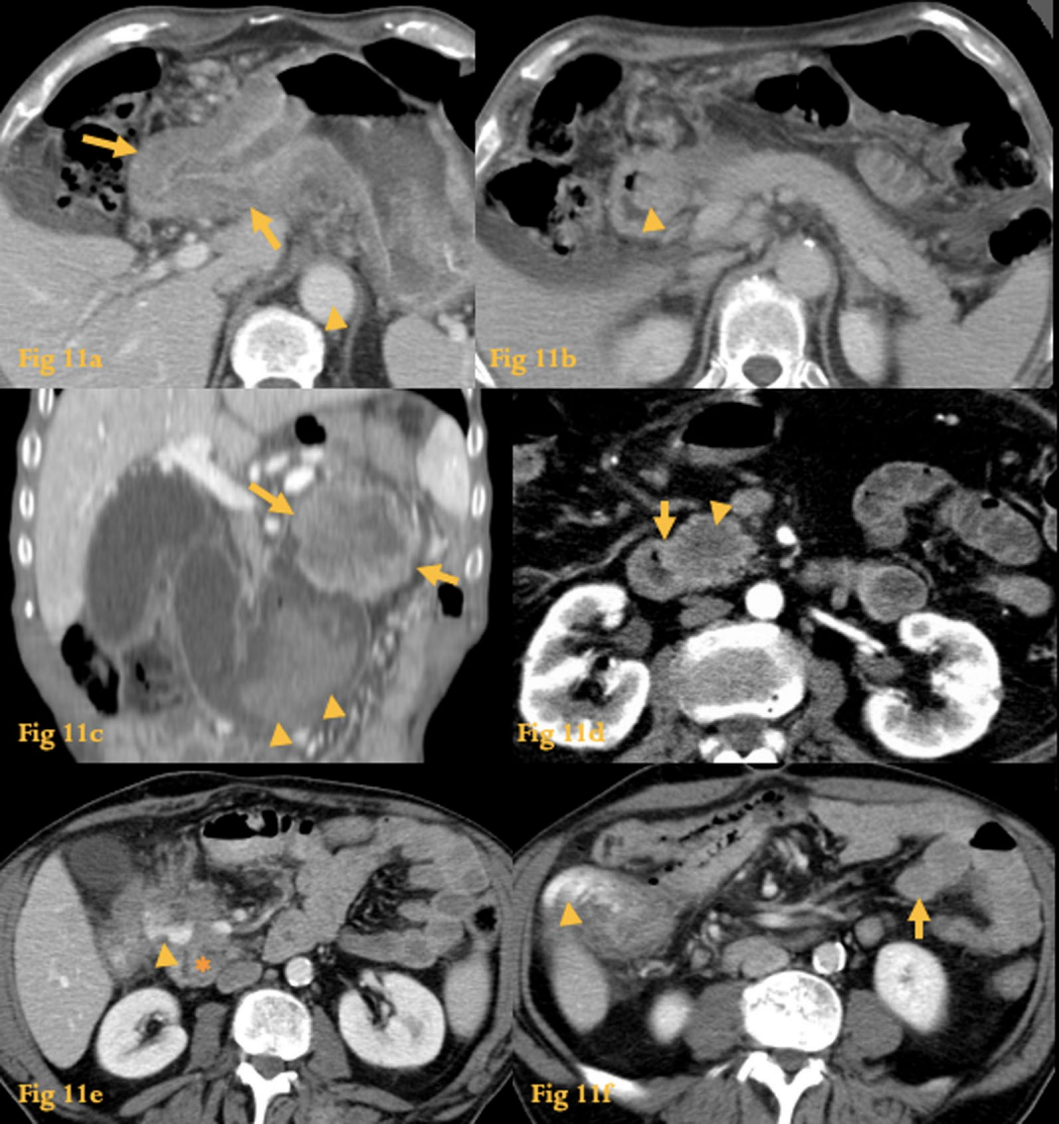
Extension from adjacent tumor. Abdominal CT (a, b) shows circumferential wall thickening of the gastric antrum (arrows) extending through the pylorus into D1 and D2, where a polypoid-like lesion (arrowhead) narrows the lumen. CT of another patient (c) shows evidence of gastric adenocarcinoma (arrows) with duodenal invasion and hemorrhage (arrowheads) inside the duodenal lumen. Abdominal CT of other patient (d) shows a hypodense soft-tissue density mass (arrowhead) which was a pancreatic adenocarcinoma with invasion of the medial wall of D2 (arrow). Abdominal CT of a patient with a neoplasm of the hepatic flexure of the colon (e, f). An undefined mass apparently involves the duodenum (asterisk) and the colon. A small amount of positive oral contrast agent (arrowhead) is present within the mass. An image obtained caudally (f) shows oral contrast agent in the colon (arrowhead) without evidence of contrast in the jejunum (arrow), a feature related to a colo-duodenal fistula
